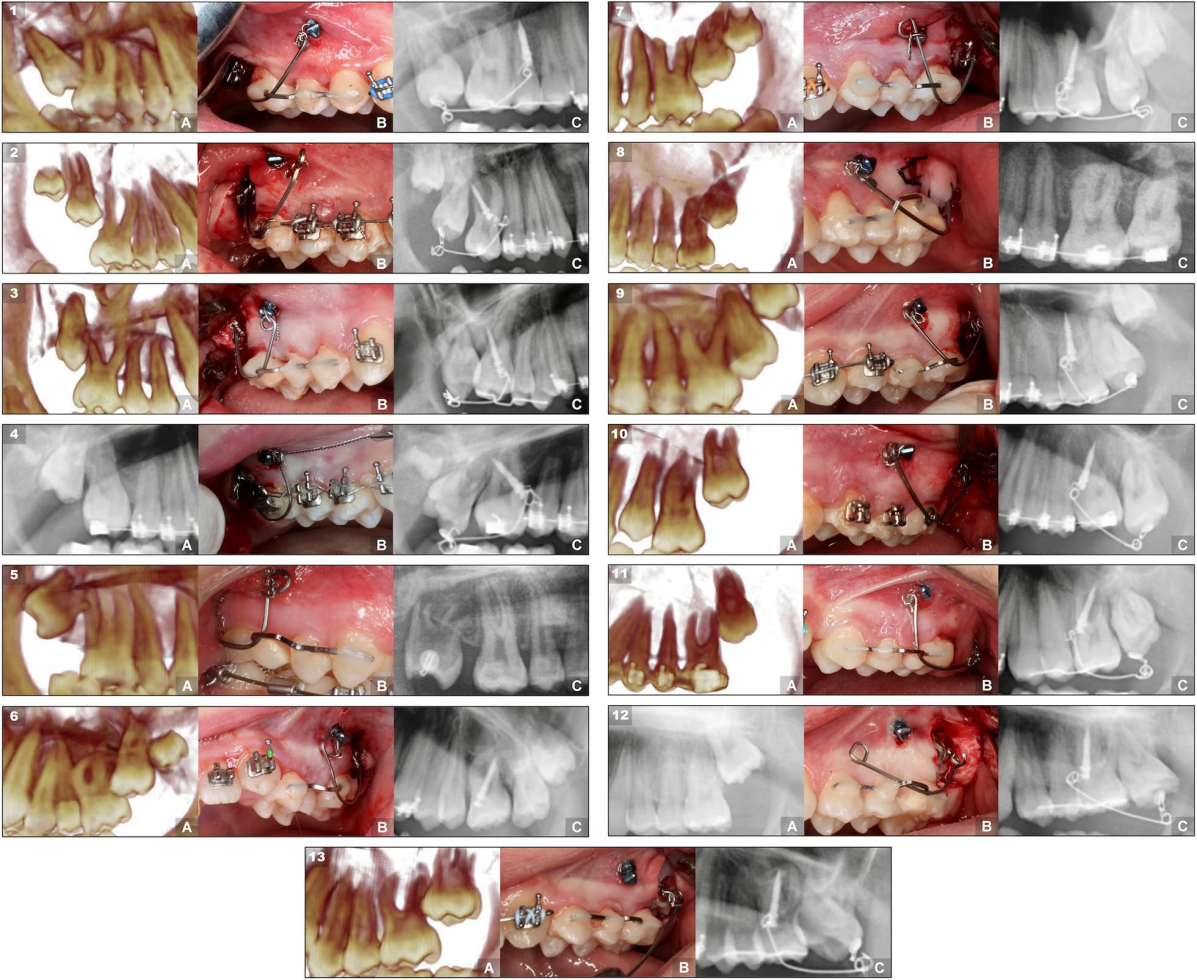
Case series of maxillary M2s treated with the miniscrew-supported pole technique. The 13 cases are organized according to their angulation, from mesial to distal, showing first the right ones and then the left ones. Each case is composed of three images that represent three moments during the treatment: a pretreatment radiograph showing the impacted or retained upper molar. b molar with the pole technique surgery carried out. c radiograph showing the final result with the molar in a correct position and angulation
